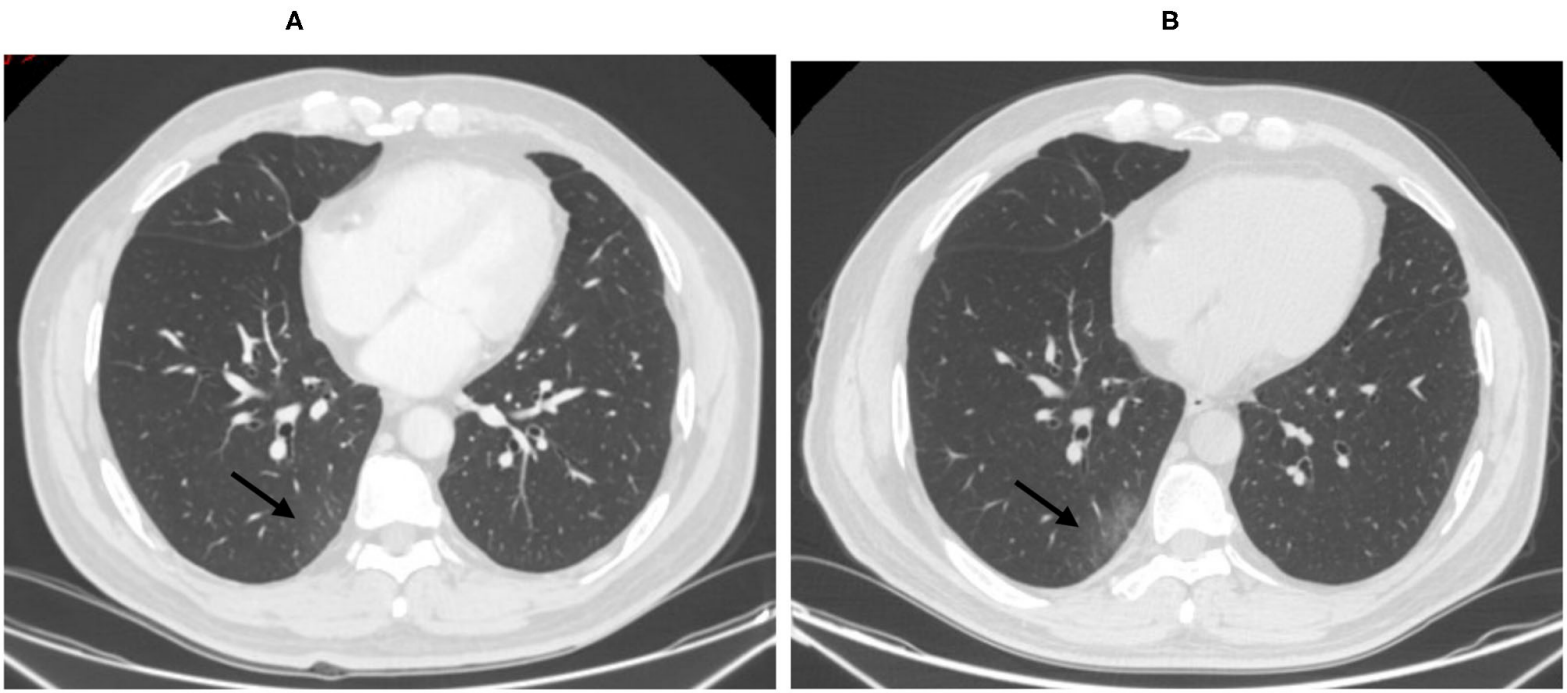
Fifty-six-year-old male with lung adenocarcinoma history. Screening CT images (B) showed subpleural bandlike areas of ground glass opacity (GGO) had been increased in size compare to the prior CT scan (A) (arrow).
